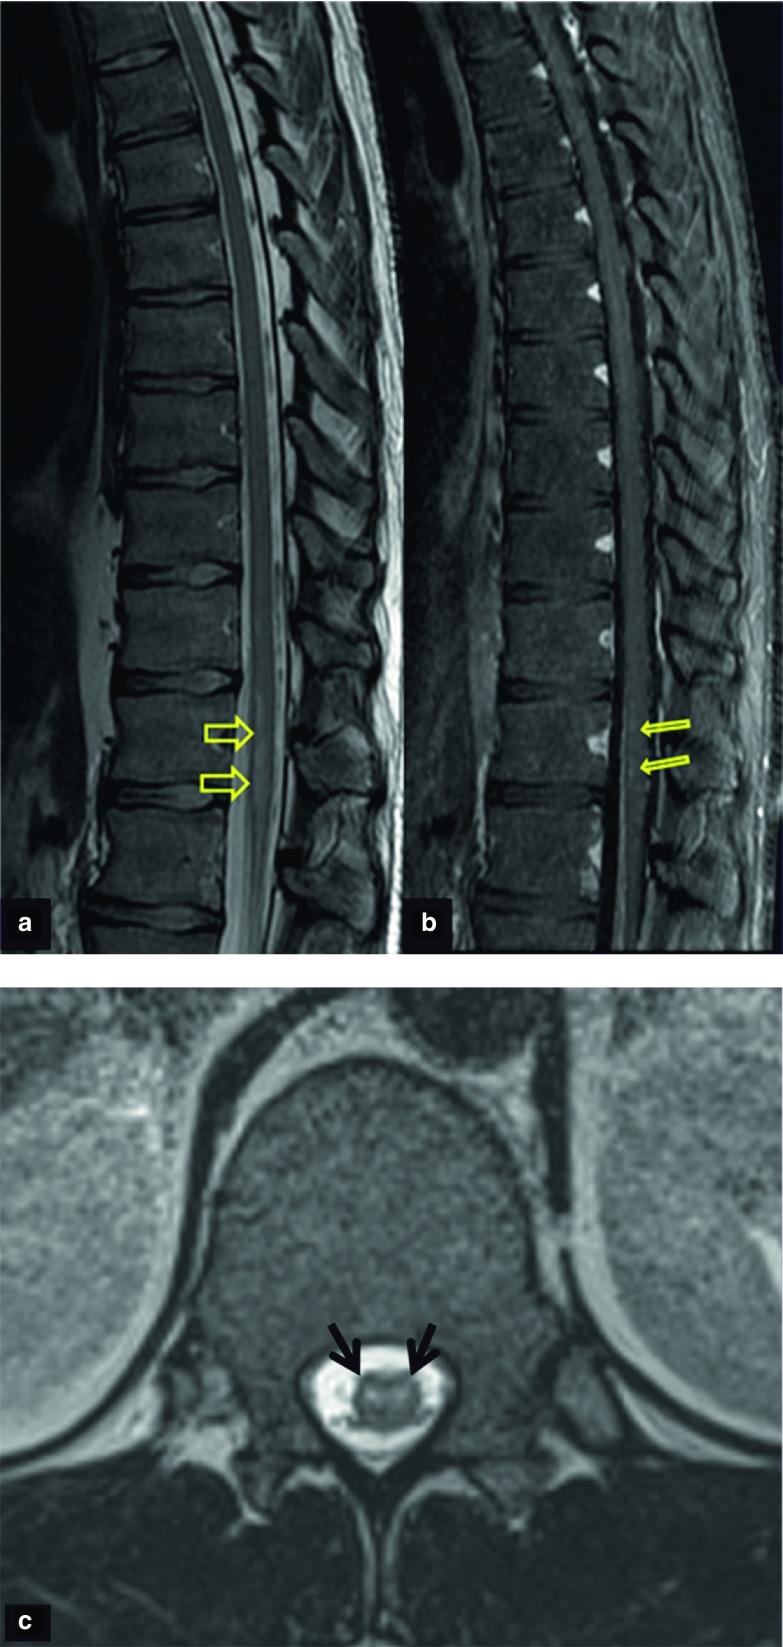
A follow-up MRI 28 days after the initial one shows more precise borders of the lesion on the *T*_2_ weighted sagittal image [arrows in (a)] and foci of enhancement on the fat-suppressed contrast-enhanced *T*_1_ weighted MR image [arrows in (b)] in keeping with subacute ischaemia. The axial three-dimensional *T*_2_ weighted MR image shows swelling and oedema within the cord at the T12 level [arrows in (c)].
